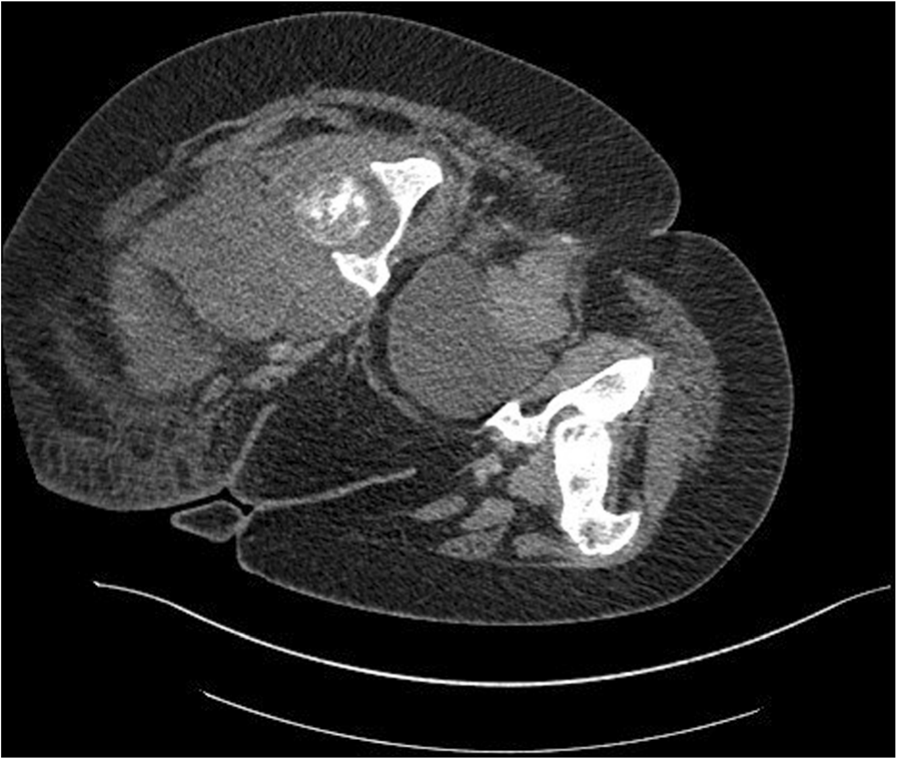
Axial computed tomography image showing a moth-eaten appearance of the femoral head
